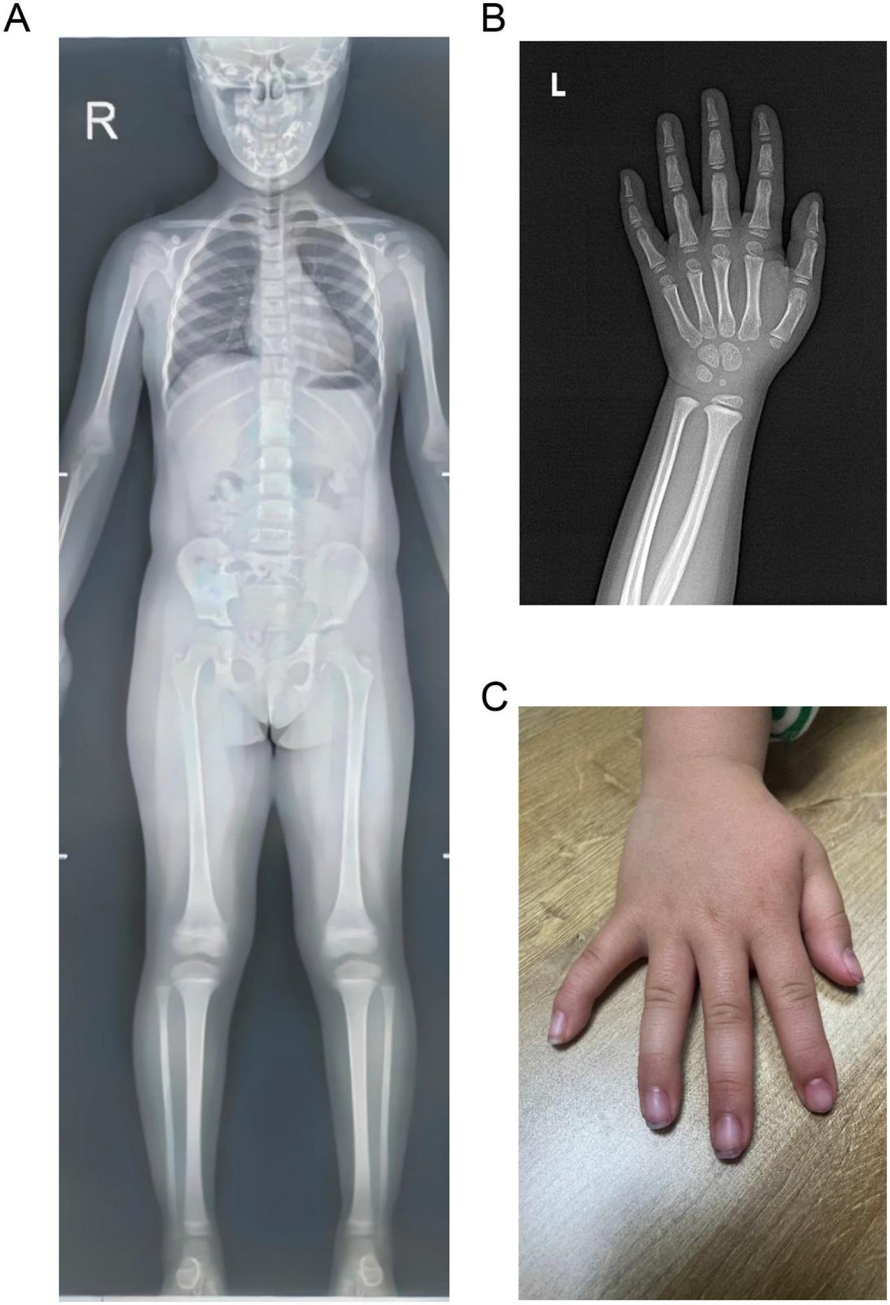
Skeletal survey images of the patient. (A) Normal anteroposterior x-ray image. (B) X-ray image of the left hand showing clinodactyly of the fifth finger. (C) Image of right hand showing clinodactyly of the fifth finger.
